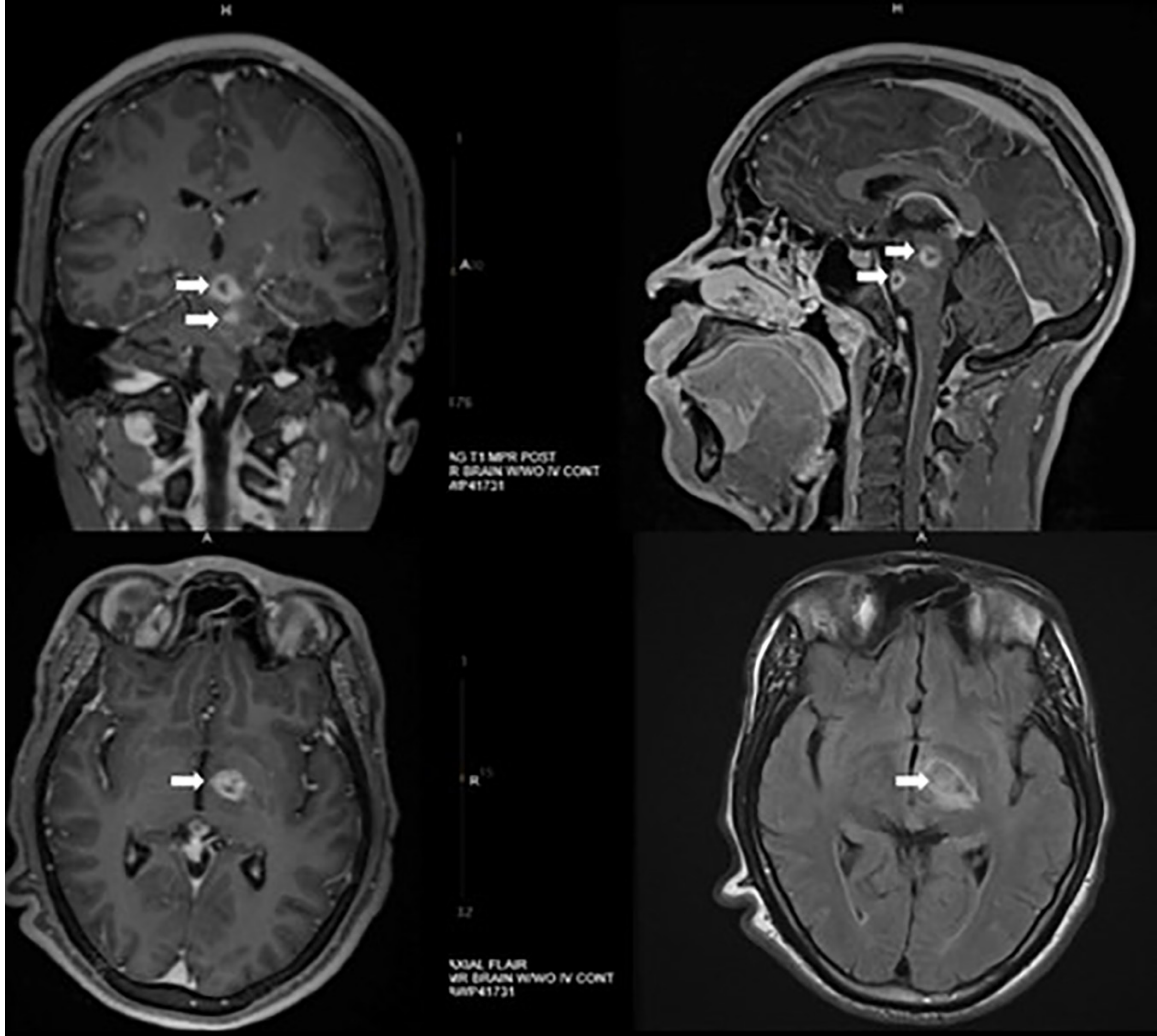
Magnetic resonance imaging of the brain with/without intravenous contrast: multifocal abnormal enhancing lesions (white arrows) in the bilateral cerebral hemispheres, left thalamus, and brainstem.
